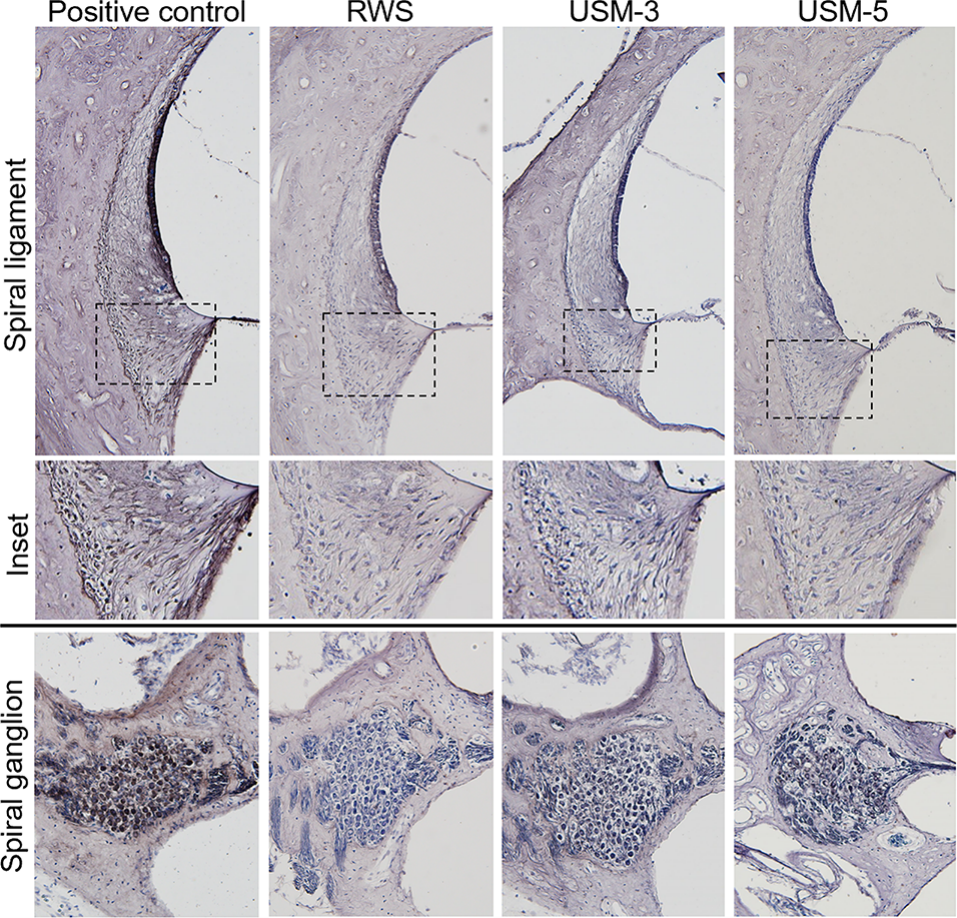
Effects of various ultrasound microbubble (USMB) courses on cell death in the target inner ear. Representative photos of the spiral ligament and spiral ganglion examined by Transferase dUTP Nick End Labeling (TUNEL) assays following round window soaking (RWS) and USMB treatment (original magnification ×200). A section treated with DNase I served as a positive control.
